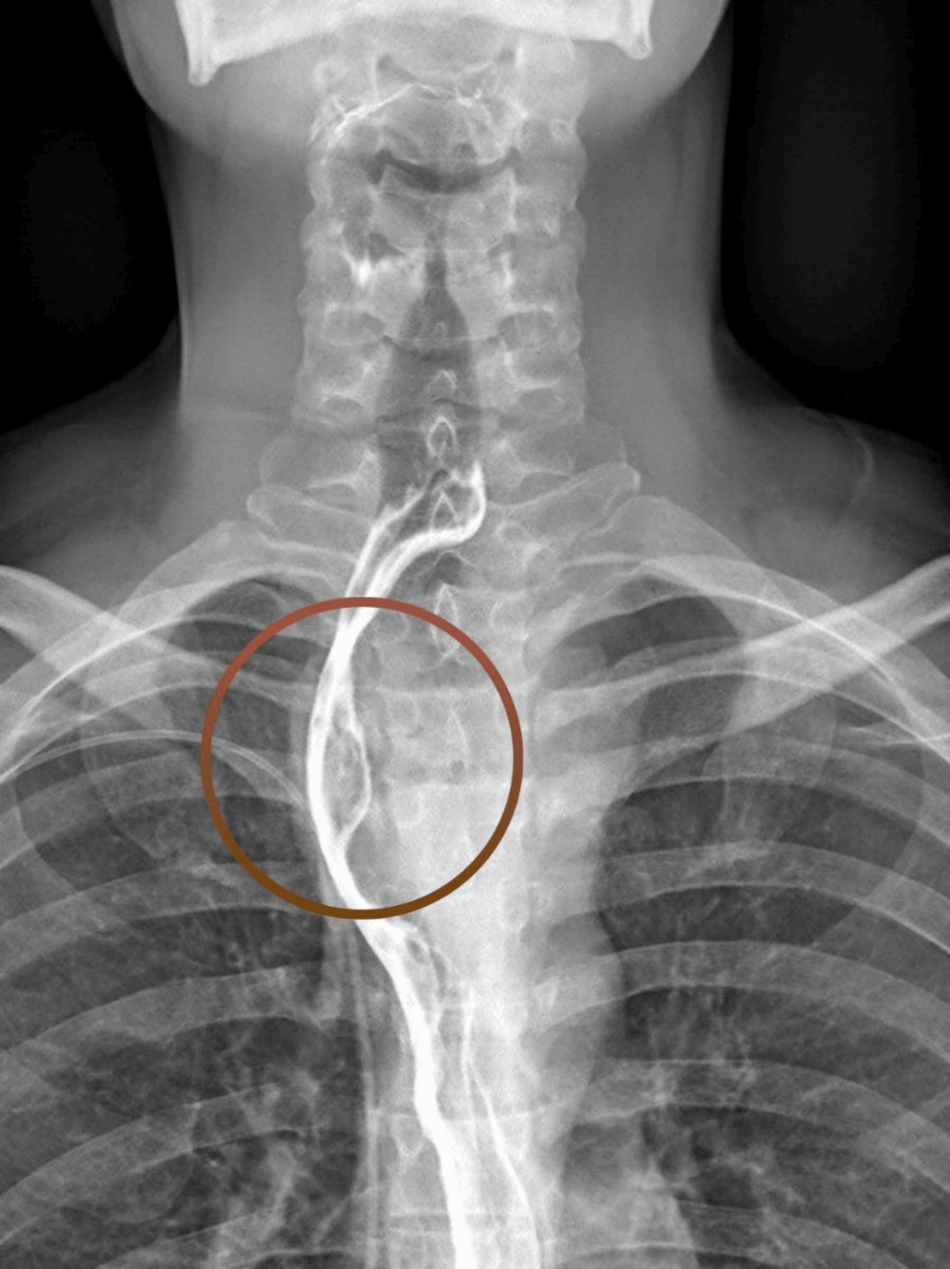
Contrast swallow fluoroscopy The esophageal mass can be seen externally compressing the esophageal lumen (brown circle). The fistulous tract between the mass and the lumen seen on CT cannot be appreciated here.
